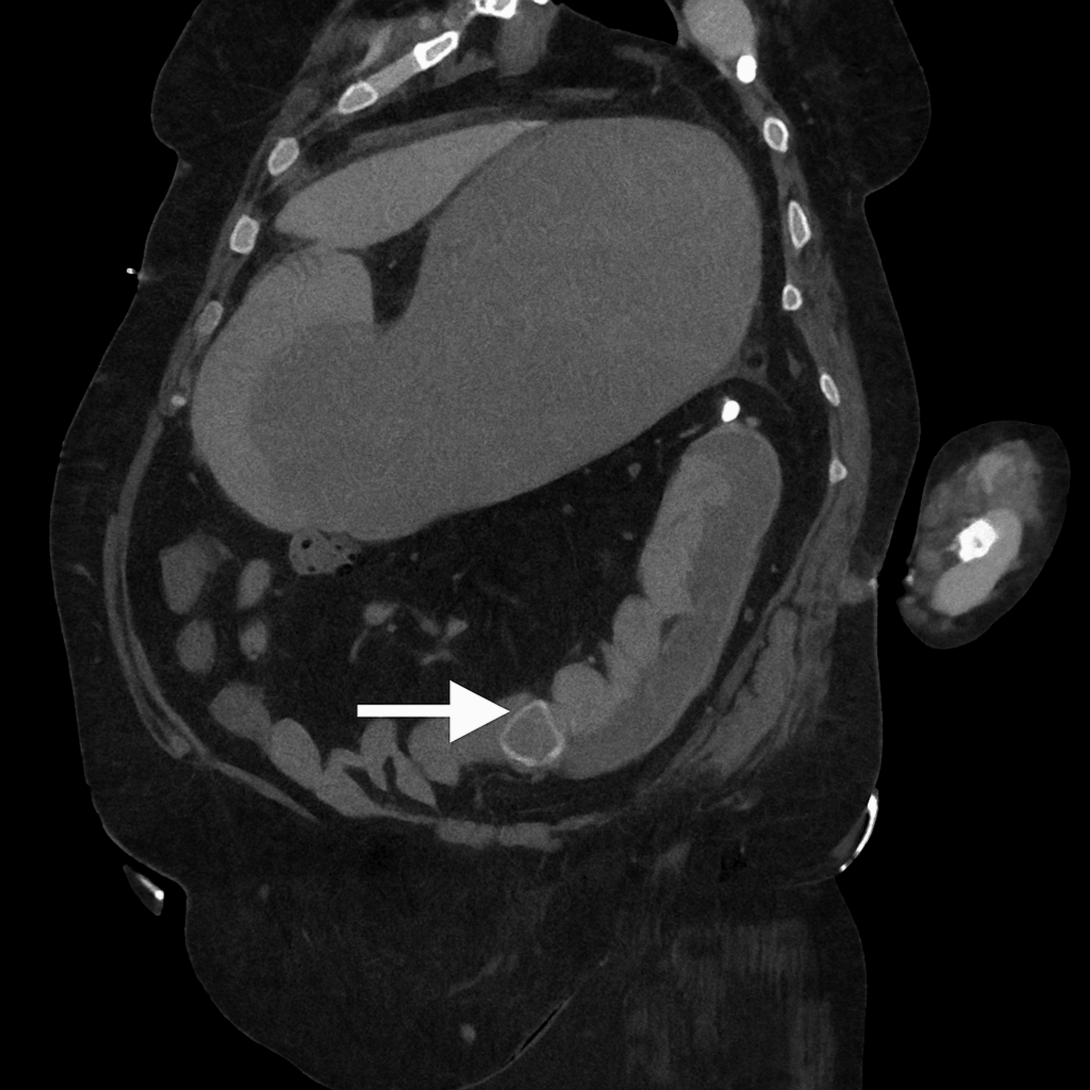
Coronal image Coronal CT image of the abdomen and pelvis demonstrating a 3.2 cm ectopic gallstone (white arrow) impacted in a loop of distal ileum, resulting in proximal small bowel dilatation consistent with gallstone ileus and mechanical obstruction.
